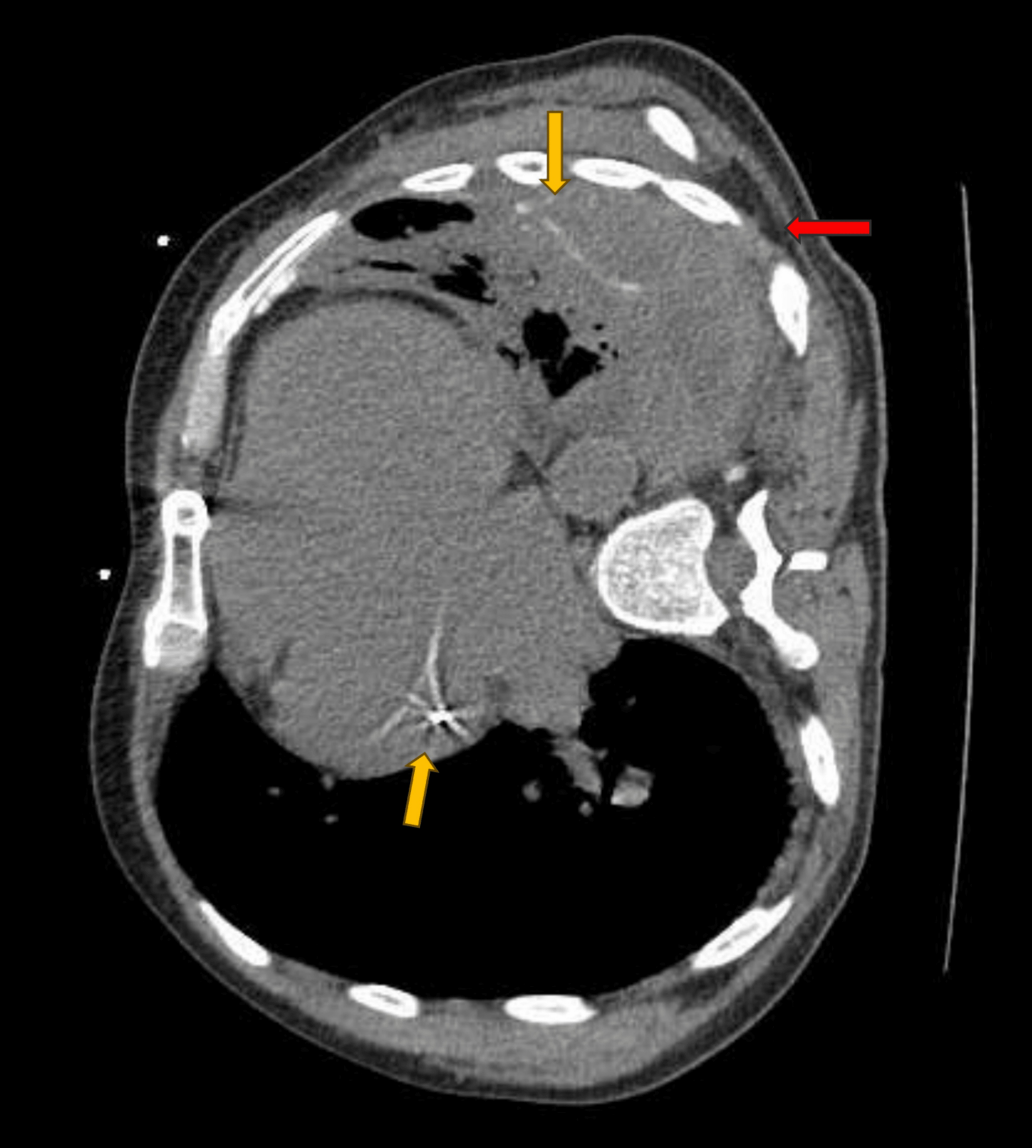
Cross-sectional view CT scan of chest without contrast Left-sided pleural thickening (red arrow) extending into the left minor fissure with irregularly shaped hyperdensities, which may represent amorphous calcifications (yellow arrows). Small-to-moderate right pleural effusion with tracking into the right major fissure. The left pleural findings are concerning for a neoplastic process.
